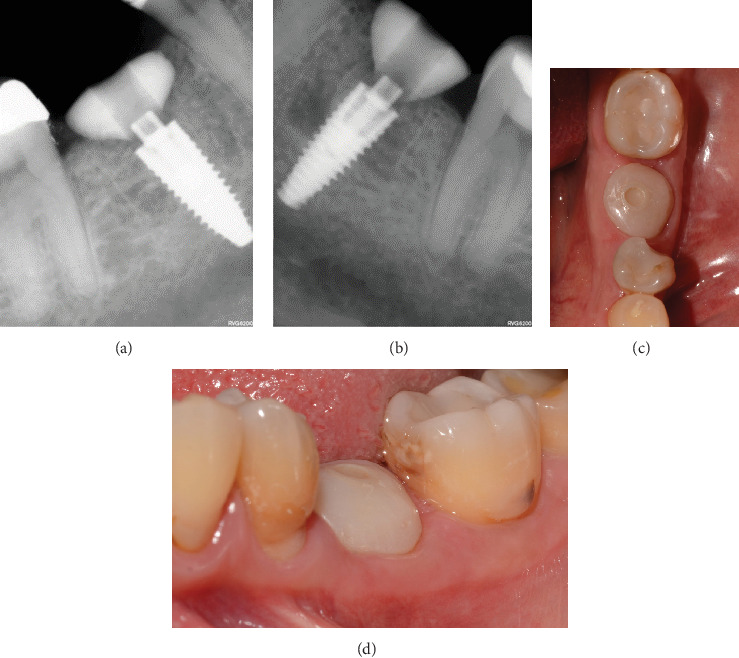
Clinical and radiographic controls: (a) postoperative periapical x-ray of Tooth 30; (b) postoperative periapical x-ray of Tooth 19; (c) clinical control 1 month after surgery, occlusal view; (d) clinical control 1 month after surgery, lateral view without any contact.
